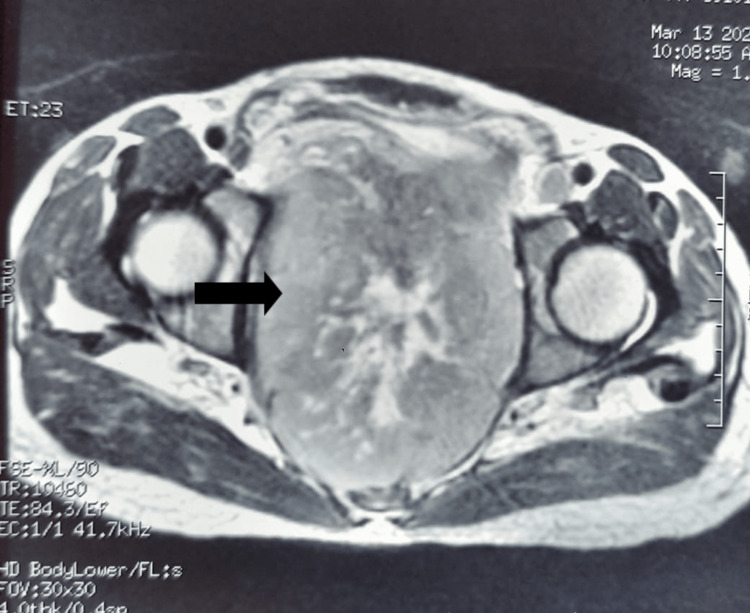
Axial T2-weighted MRI image The image shows a locally advanced solid necrotic pelvic mass (black arrow).
